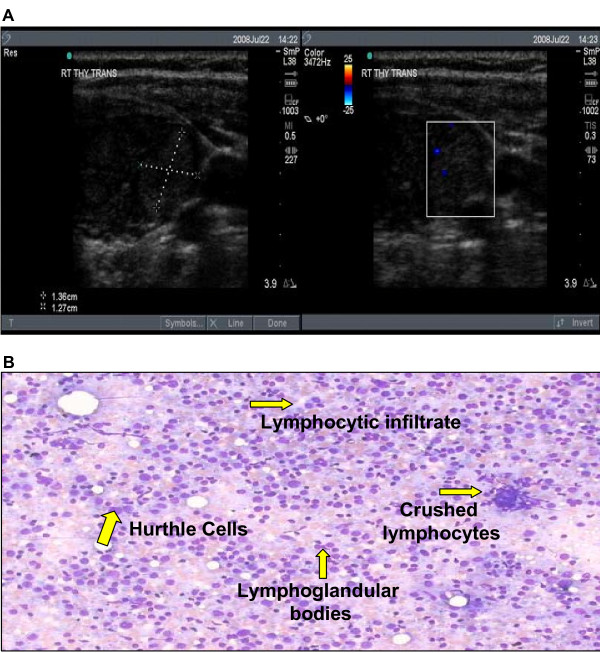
A. Ultrasound image of euthyroid Hashimoto thyroiditis. A representative ultrasound is shown of an asymptomatic, euthyroid patient's thyroid nodule cytologically diagnosed Hashimoto thyroiditis. Note the minimal color flow Doppler identified within the nodule. B. Cytopathology. Cytological characteristics of a thyroid nodule with typical diagnostic features of Hashimoto's thyroiditis (i.e. lymphocytic infiltrate, Hurthle cells, lymphoglandular bodies and crushed lymphocytes).
